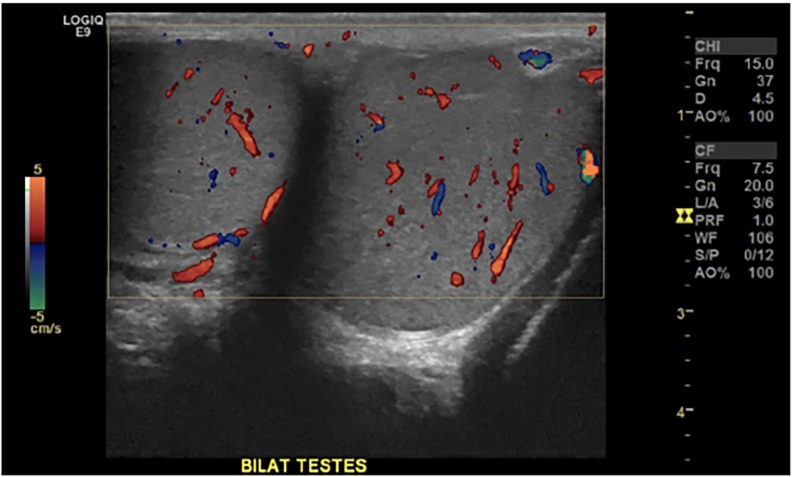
The patient’s normal scrotal ultrasound.
